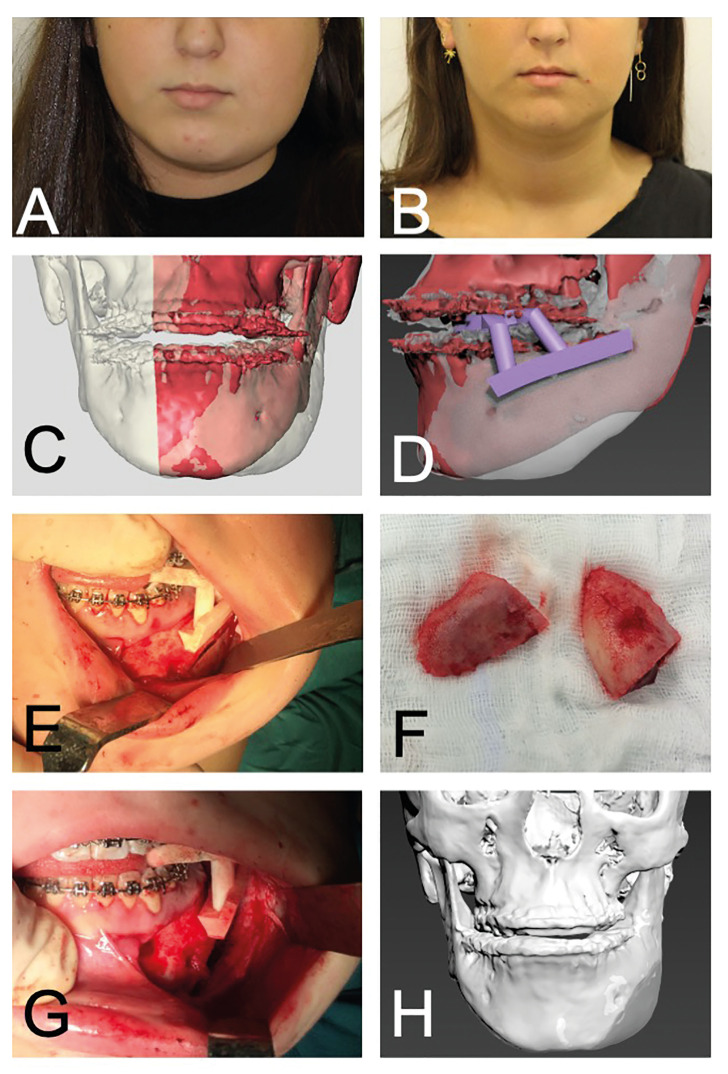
A 13-year-old patient with DSO/TP of the left mandible. (A + B) Pre- and post-operative pictures, on which a decrease in swelling of the left mandible can be observed. (C + D) Three-dimensional reconstruction (3D) of CBCT-scan on which the osseous swelling of the left mandible can be seen. In red a mirrored model of the mandible to determine the extent of asymmetry and to determine the resection osteotomy with matching patient-specific surgical guides. (E) Peri-operative image after incision with visualization of the mental foramen and placement of the occlusion-based patient-specific surgical guide. The osteotomy-lines prepared with a saw can be seen. (F) The removed bone parts. (G) Picture taken intra-orally after removing the bone parts with the patient-specific surgical guide still inside. (H) 3D reconstruction of CBCT-scan pre- (transparent) and postoperative, showing a more symmetrical appearance.
